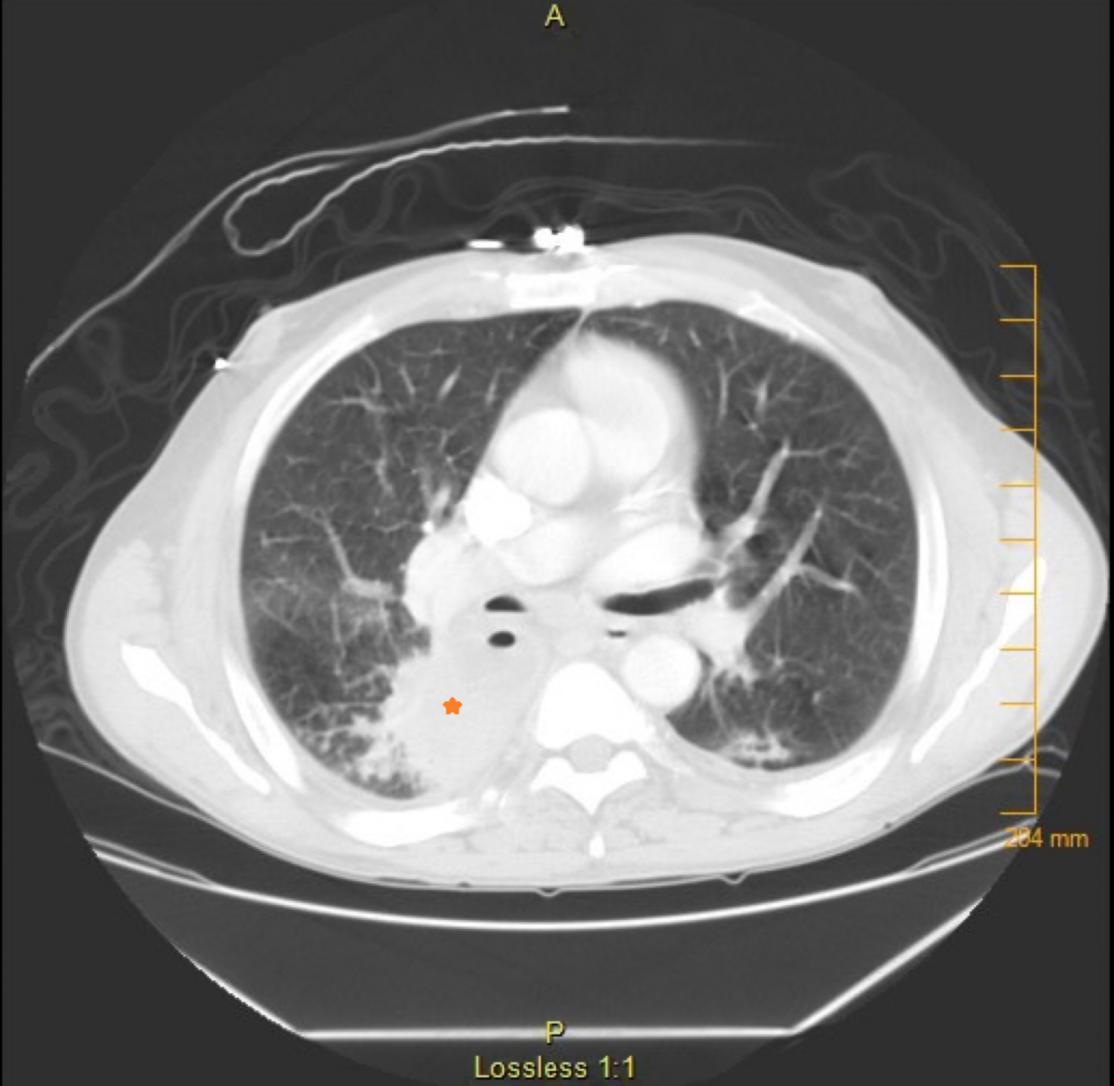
CT thorax with contrast showing a cavitary lesion in the right lower lung lobe. Orange star pointing to the consolidation. CT: computed tomography
